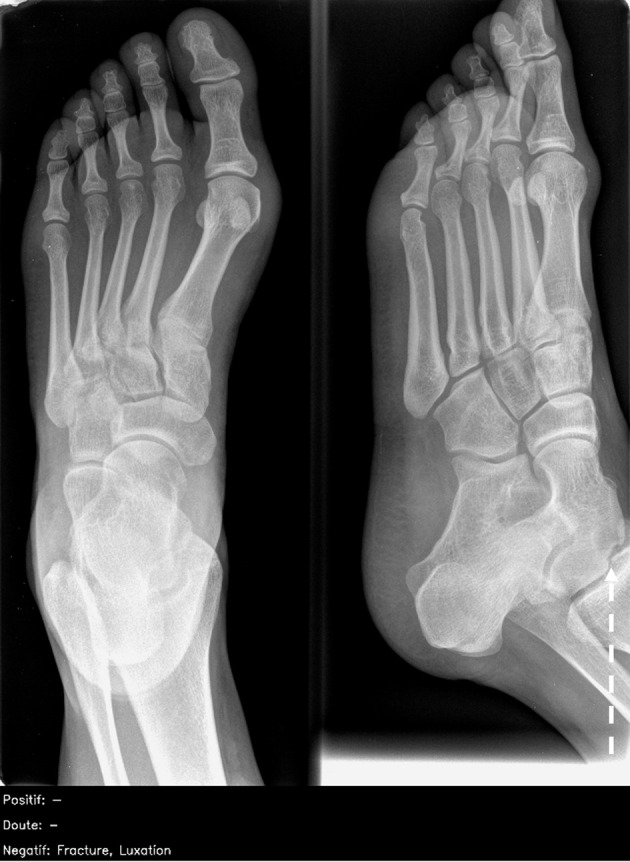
Dorsoplantar and oblique views of abnormal right foot radiograph in adult showing osteochondral defect at talar dome (white dashed arrow). This finding was missed by all 26 radiologists and also artificial intelligence candidate (false negative) and was particularly challenging. French translation: doute=doubt; luxation=dislocation; negatif=negative; positif=positive
